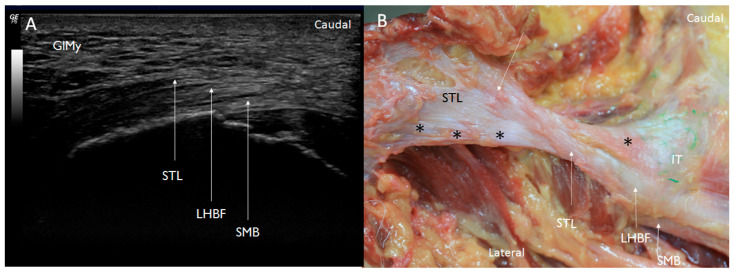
(A) Ultrasound view at the long axis showing that the left sacrotuberous ligament (STL) is seen as a hyperechogenic structure that continues caudally with the tendon of the long head biceps femoris (LHBF). Also, the tendon of the semimembranosus muscle is located as a hyperechogenic structure under LHBFB. GlMy: Gluteus maximus muscle (B) Anatomical dissection showing that the fibers of the left STL cross between them with a lambda (λ)-shaped bifurcation. Then, the superficial and medial side of the STL (white arrow) goes on caudally with the LHBF. Moreover, it is possible to observe that the lateral and the deeper part of the sacrotuberous ligament inserts at the ischial tuberosity (black *), showing an x shape. The deeper layers of the ligament (black *) insert at the ischial tuberosity (IT).
